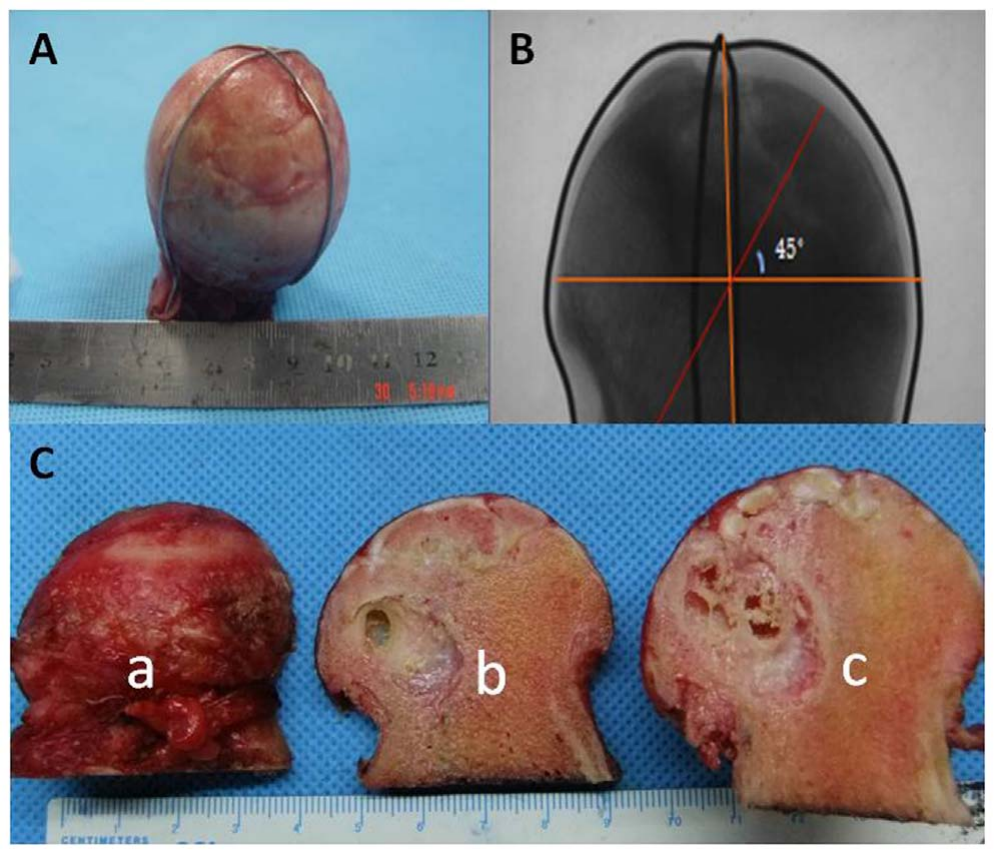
Cutting method of the femoral heads. The femoral heads were marked with an iron wire in the coronal plane and subjected to X-ray to confirm that all of the samples showed similar processes (A, B). The samples were divided into three parts: *a* for paraffin sections, *b* for real-time PCR and Western blotting, and *c* for undecalcified tissue sectioning and nanoindentation (C, D).
